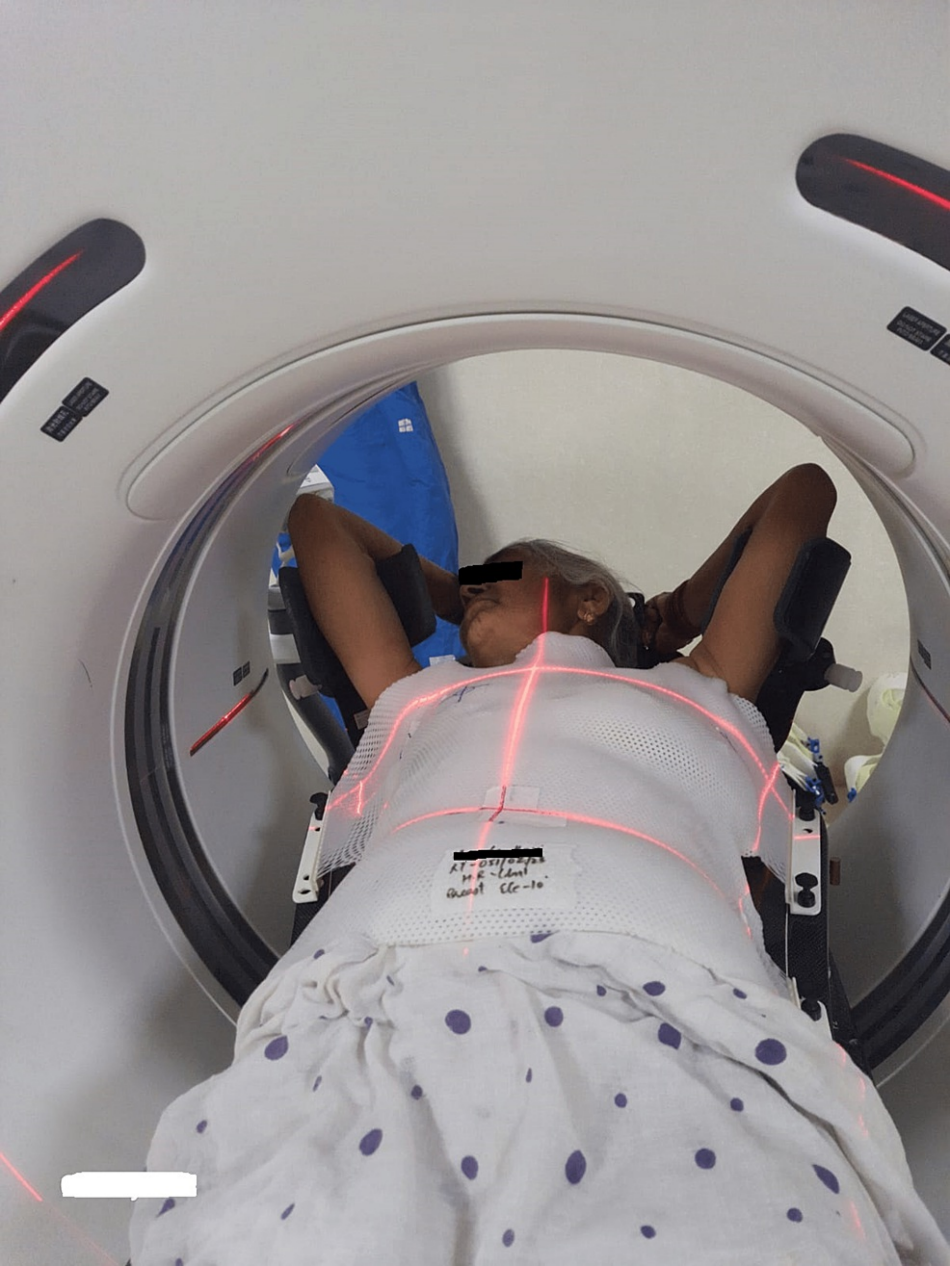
Computed tomography (CT) image acquisition The CT scan images acquired on Optima CT-580 RT (GE Healthcare, India)
